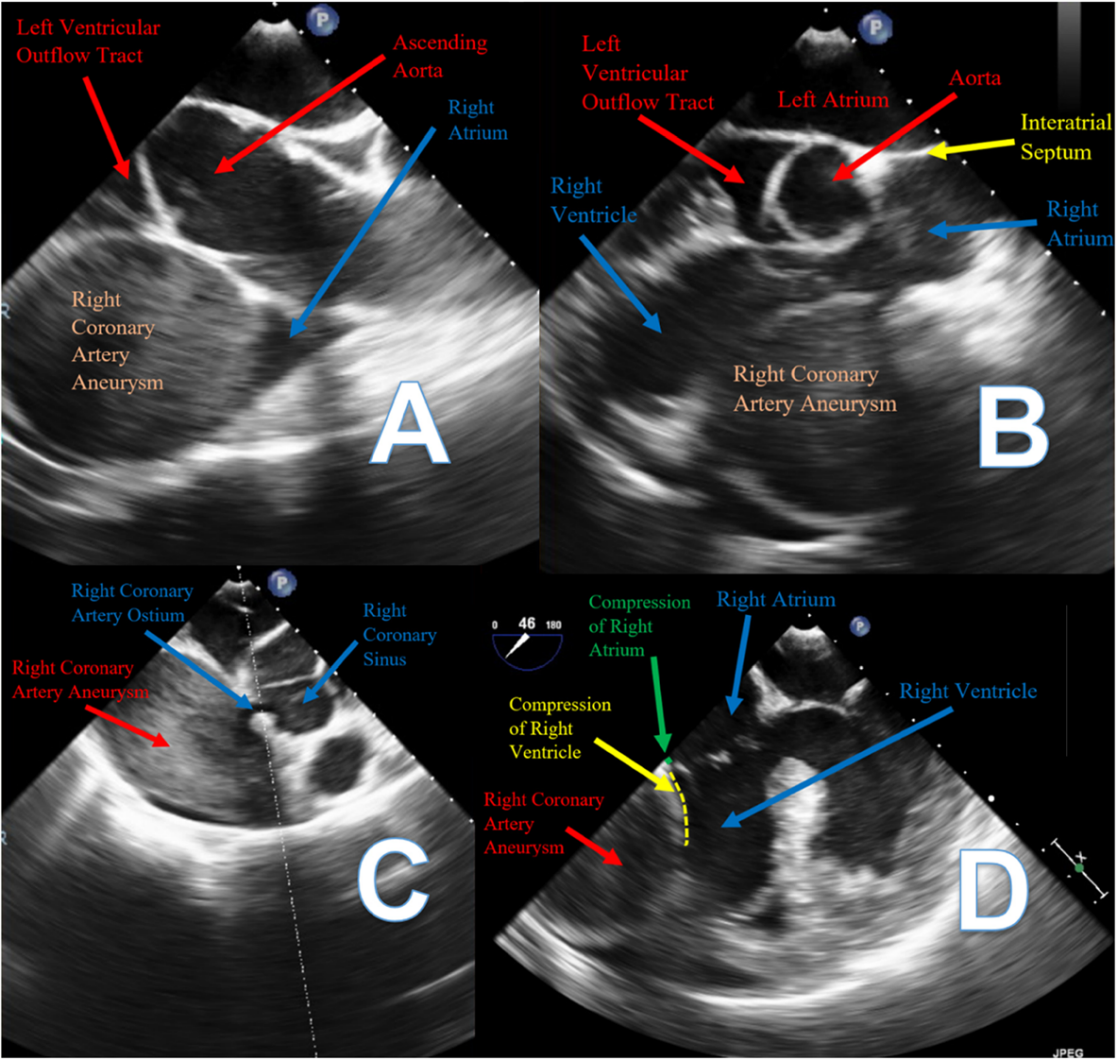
Intraoperative Transesophageal Echocardiograms. A Turbulent flow within the giant RCAA. B Extrinsic compression of the right ventricle by the giant RCAA. C Ostium of the giant RCAA from the right coronary sinus with turbulent flow in the aneurysm. D Extrinsic compression of the right atrium and the right ventricle by the giant RCAA
